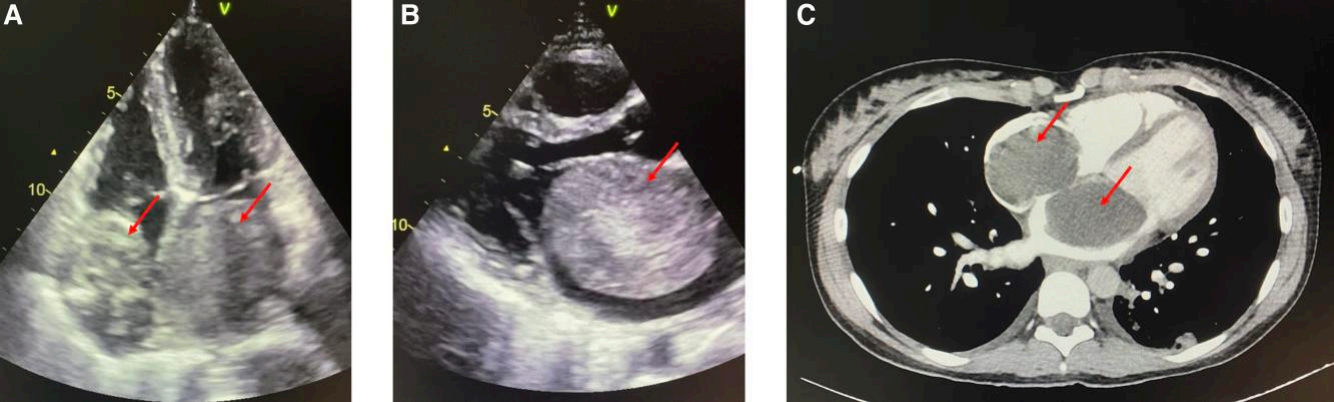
(*A*–*C*) Pre-operative imaging of the biatrial myxoma. (*A*, *B*) Transthoracic echocardiography (*A*: apical four-chamber view, *B*: parasternal long axis view), (*C*) CT scan, the arrows indicate the myxoma.
